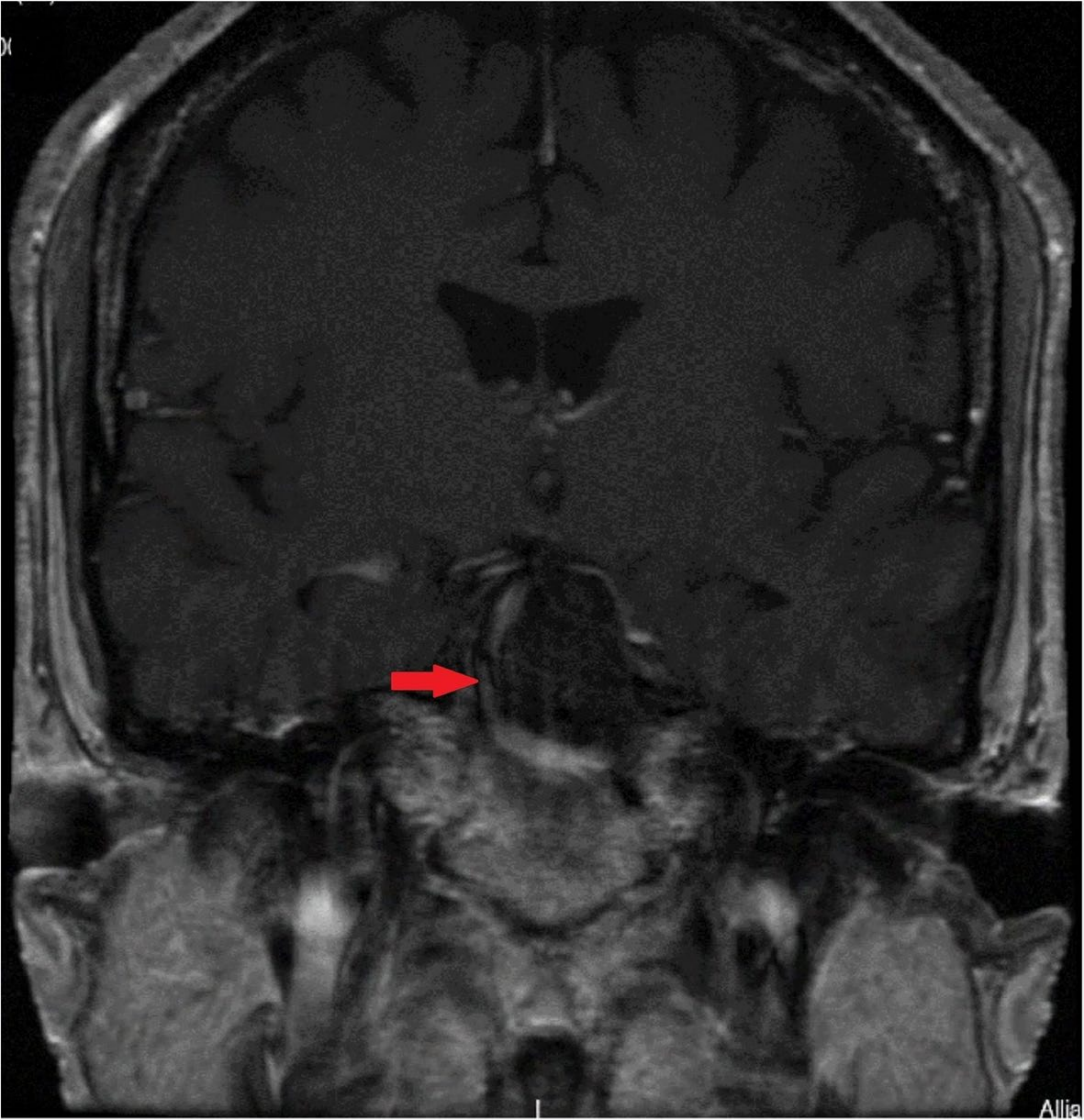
T1 coronal MRI pituitary 6 months post-hypophysectomy showing 13 mm residual tumour
